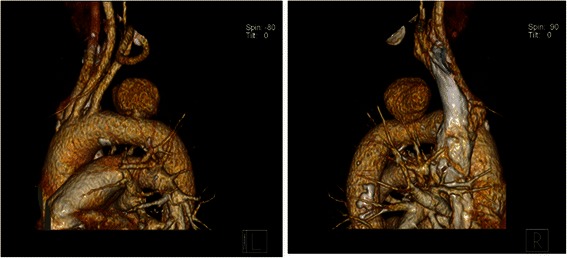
Three-dimensional computed tomography images for the shape of pseudoaneurysm on aortic arch, with a size of 2.9 × 4.3 × 2.6 cm. L: left side, R: right side
